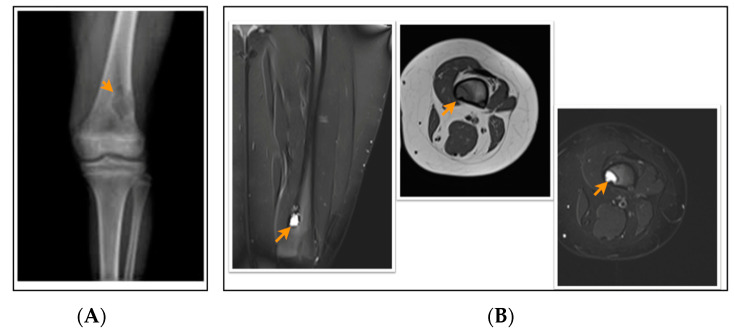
A 12-year-old patient with a fibrous cortical defect around the knee. (A) Anteroposterior direct X-ray of a 12-year-old patient shows a lytic lesion with a sclerotic rim in the distal posteromedial metaphysis of the femur consistent with FCD. (B) Coronal and axial proton density and axial T1-weighted MR sections of the posteromedial left femur show a lesion consistent with a fibrous cortical defect. This lesion was classified as stage B according to Ritschl’s classification, because of lobulated contour. Clinically, IPAQ (International Physical Activity Questionnaire): Inactive, Screen Time: 5 h, 21-Numbered Circle VAS (21-VAS): 4, VAS (Visual analogue scale): 4, 21-Numbered Circle Activity Scale (21-NCAS): 4.
